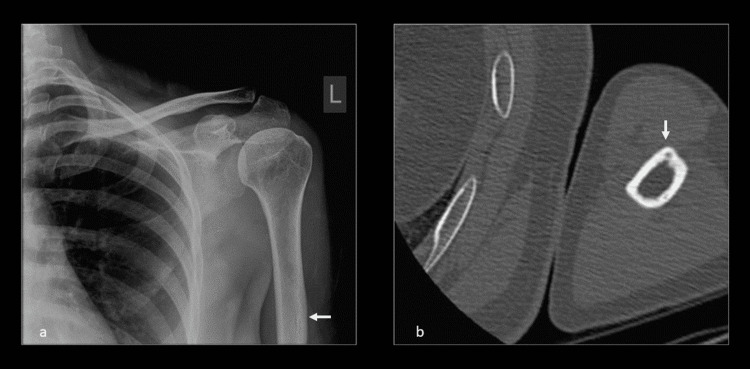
X-ray and CT evaluation of the left shoulder. (a) Frontal radiograph of the left humerus shows endosteal and cortical thickening laterally at the level of the deltoid insertion. (b) Axial CT of the left humerus shows thickened lateral cortex and radiolucent center of the lesion. CT, computed tomography
